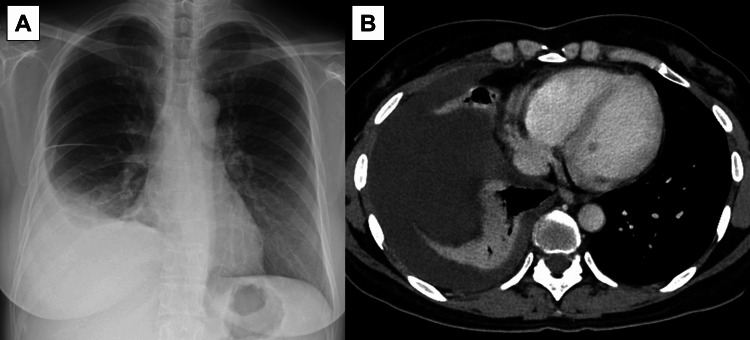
Chest radiography (A) and computed tomography scans (B) showed a large amount of right pleural effusion without any lung lesions
